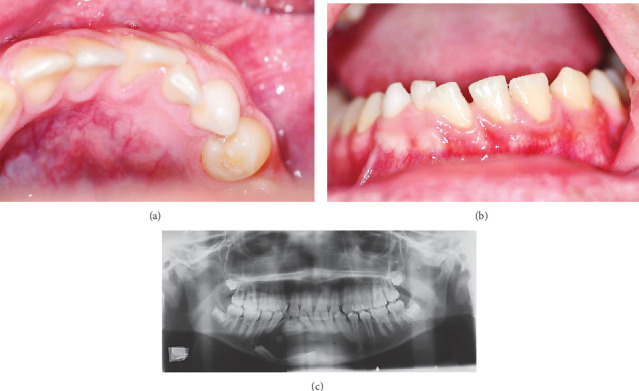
(a) Occlusal view revealing vestibular and lingual volume enlargement with intact covering mucosa. (b) Persistence of deciduous teeth and displacement of Teeth 4.1, 3.1, 3.2, and 3.3. (c) Panoramic radiograph: a well-defined radiolucent extending from Teeth 3.4 to 4.5, with calcified elements within the lesion. Tooth 4.3 displaced caudally.
